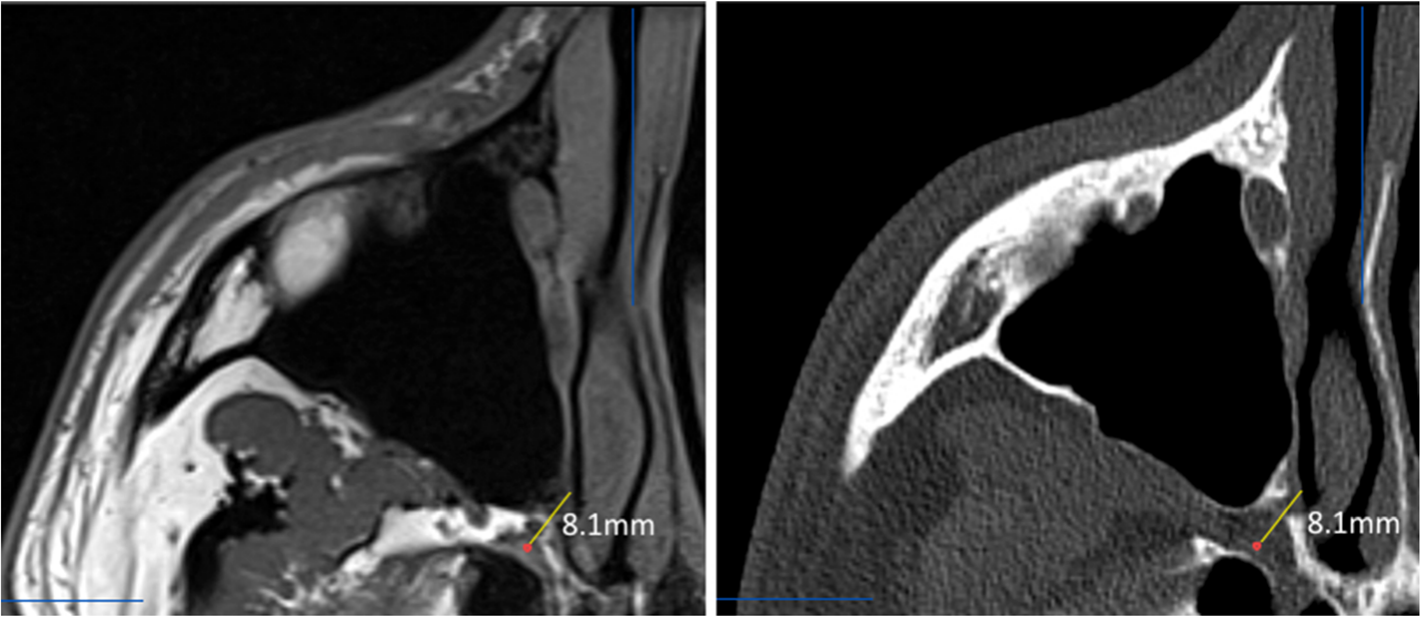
Axial images through the SPG in one of the patients. Left: T1 weighted MRI. Right: CT scan. Both images show the same anatomical plane. The SPG (red dot) is first localized in the MRI scan and the closest point of the nasal mucosa through the SPF is localized in fusioned CT images. In this example, the distance was 8.1 mm (yellow line). Notice the typical crescent form of the SPG anterior to the opening of the Vidian canal
